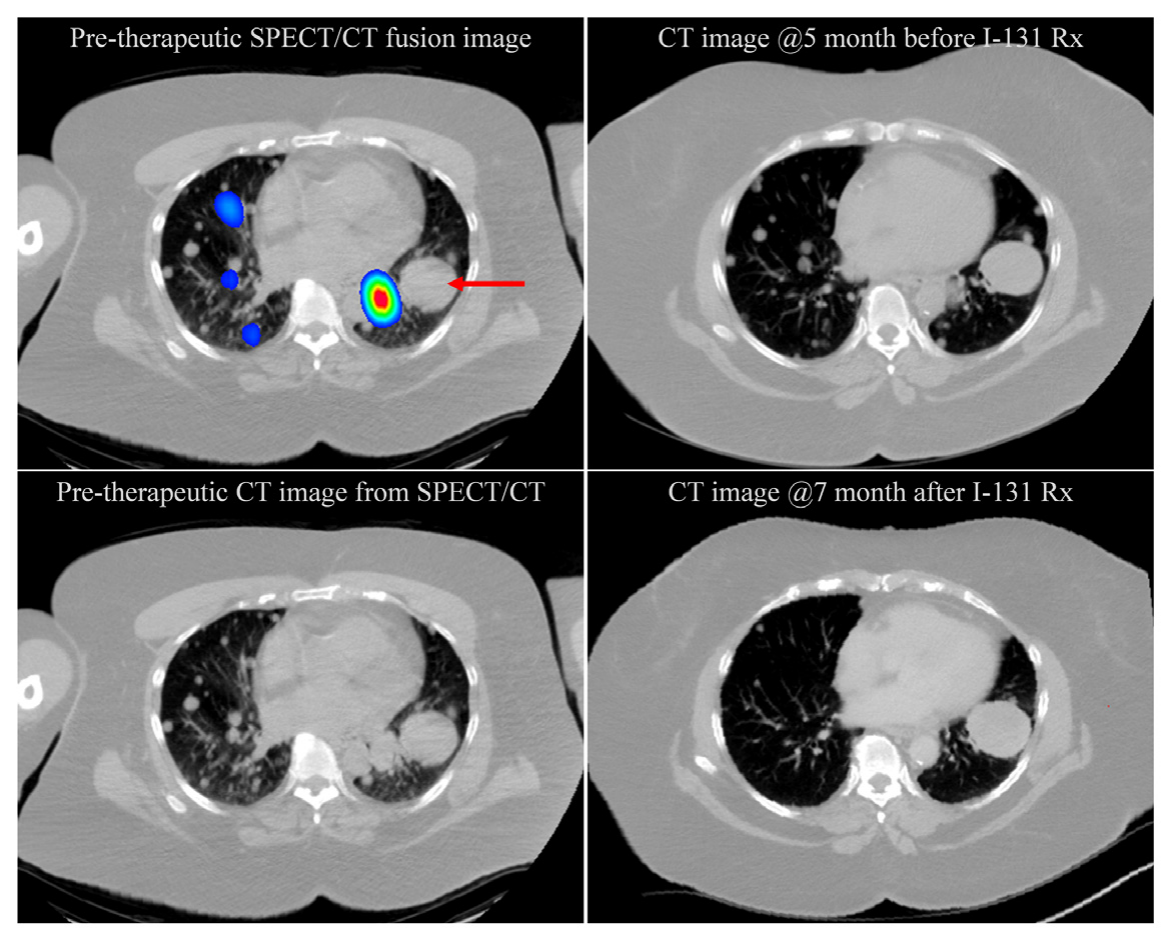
Pretherapeutic ^123^I SPECT/CT image and CT chest images prior to and after ^131^I therapy. Pretherapeutic ^123^I SPECT/CT fusion image shows heterogeneity in radioactive iodine (RAI) avidity among metastatic lung lesions, with the largest lesion being RAI non-avid (red arrow). Seven months after ^131^I treatment (121 mCi), ~160 lesions that include RAI-avid and RAI non-avid lesions were no longer detectable.
